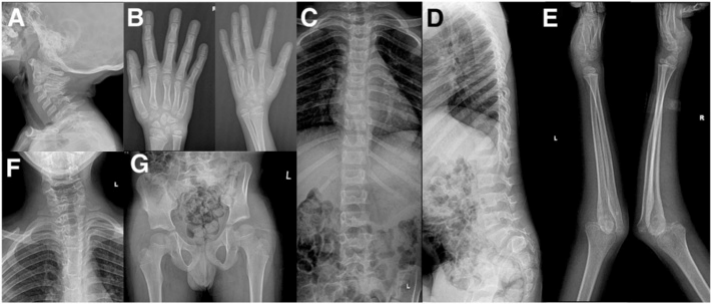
Radiographs of patient 1 at the age of 8 years. He showed normal cervical spine without instability (a), supernumerary carpal bones (b), normal thoracolumbar spine (c and d), bilateral elbow dislocation (e), cervical-dorsal scoliosis (f), and normal hip joints (g)
